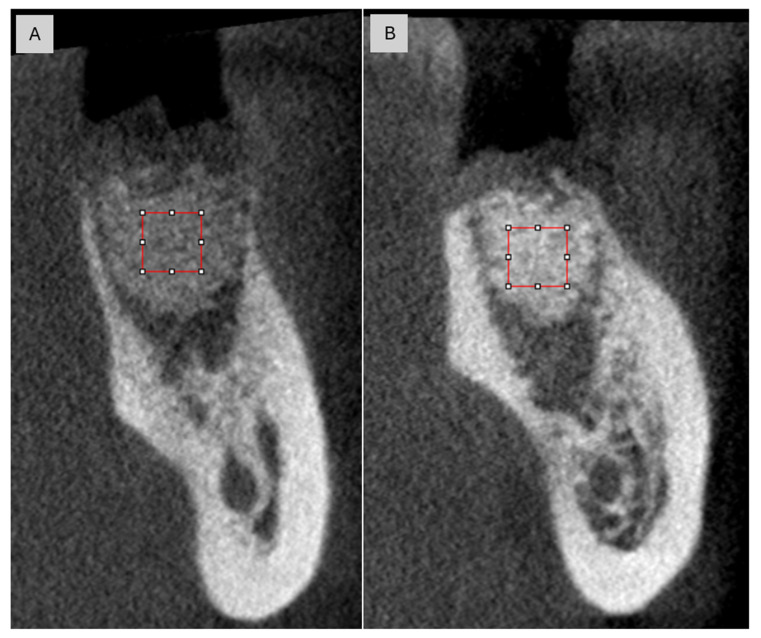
Representative images showing the selection of the region of interest (ROI) for radiographic bone density analysis. (A) Sagittal cross-section image obtained 7 days after the procedure with the ROI marked (red square) within the augmented alveolar ridge (area = 3.75 mm^2^). (B) Corresponding image obtained 6 months after augmentation, with the ROI placed in the same region and with the same area (3.75 mm^2^) for comparative density analysis.
